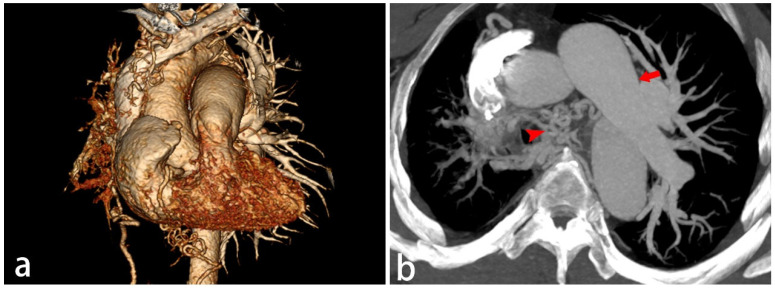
A 18-year-old male with proximal interruption of the right pulmonary artery. Volume-rendered CT angiography (a) and maximum intensity projection (MIP) image of CT angiography (b) show that the left pulmonary artery is in the normal position ((b), red arrow). The aortic arch is left-sided. Multiple dilated and tortuous systemic collateral vessels are seen in the mediastinum and right hilum ((b), arrowhead).
